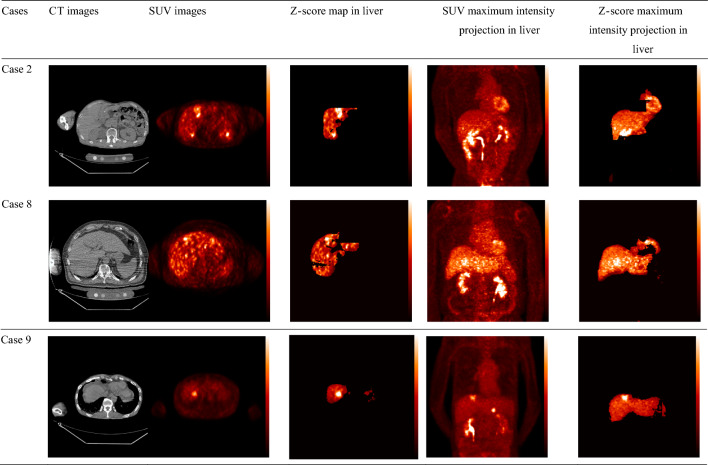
A visual representation of the standardized uptake value and Z-score in positron emission tomography/computed tomography images to understand the differences between them. We present three cases as examples
